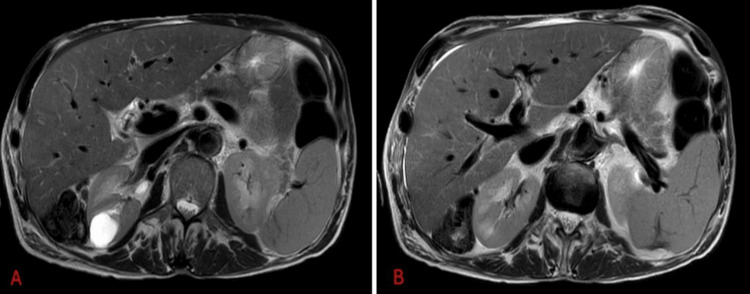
MRI of the liver A: T1 weighted; B, T2: weighted; A/B: Liver with slight heterogeneity of parenchymal texture, related to suspicion of hepatitis. Multiple liver hilar lymphadenopathy, with short axes that do not exceed 7 mm. Splenomegaly, measuring the spleen 174 mm in the greatest axis. No other relevant changes. MRI, magnetic resonance imaging
